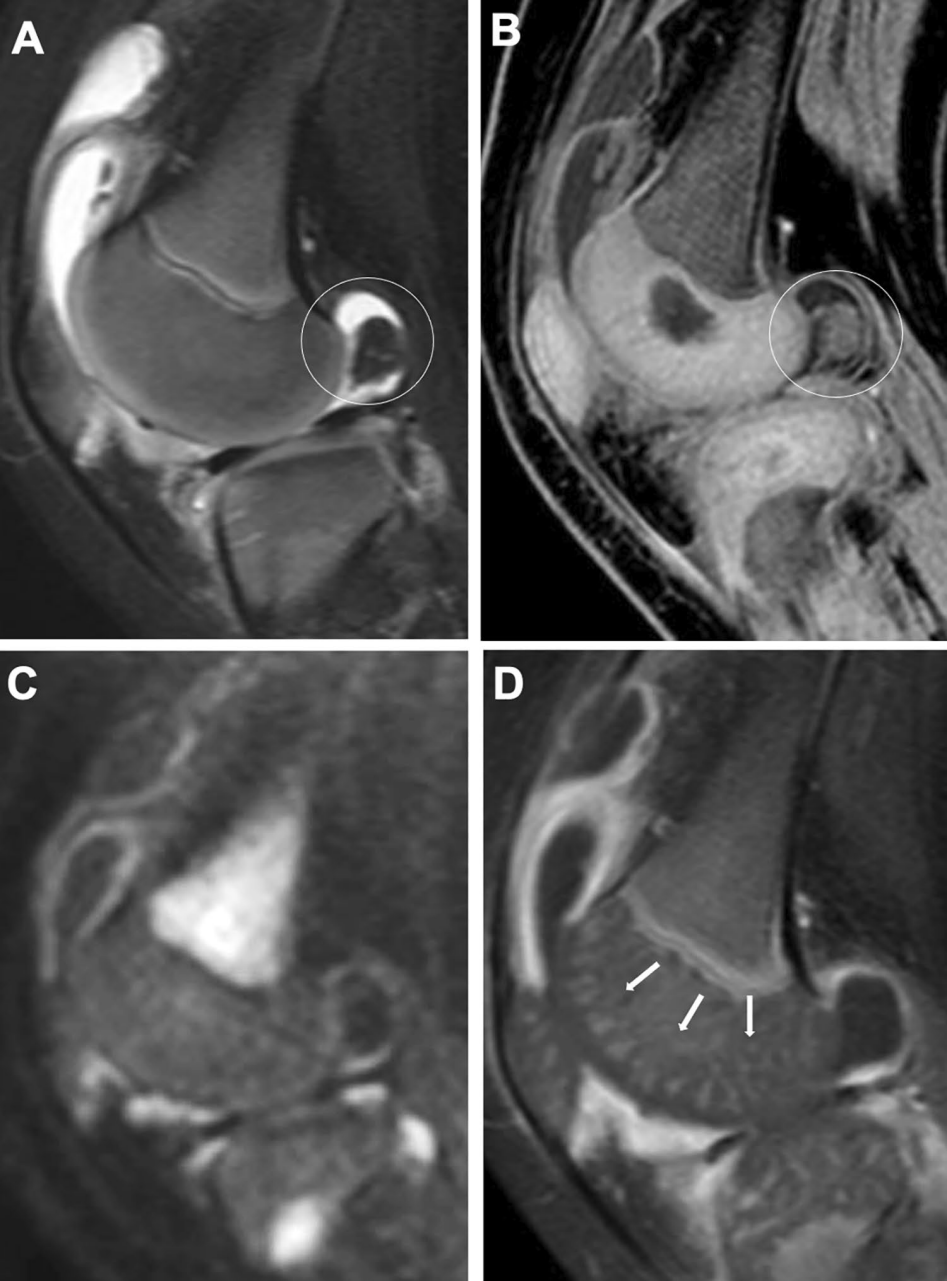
MRI for synovitis. A–D. A 1-year-old infant with painful left knee. Fat-suppressed T2-weighted (A) and water selective gradient echo sagittal images (B) showed a large pannus in the posterior fossa (circles, A, B). The articular cartilage was differentiated from the epiphyseal cartilage on both sequences. Diffusion-weighted (C) and Gd-enhanced T1-weighted sagittal images (D) showed high intensities of the synovium, indicative of active synovitis, whereas the popliteal pannus appears black and indistinguishable from joint fluid on these sequences. Vascular structures in the epiphyseal cartilage were well seen on Gd-enhanced image (arrows, D)
